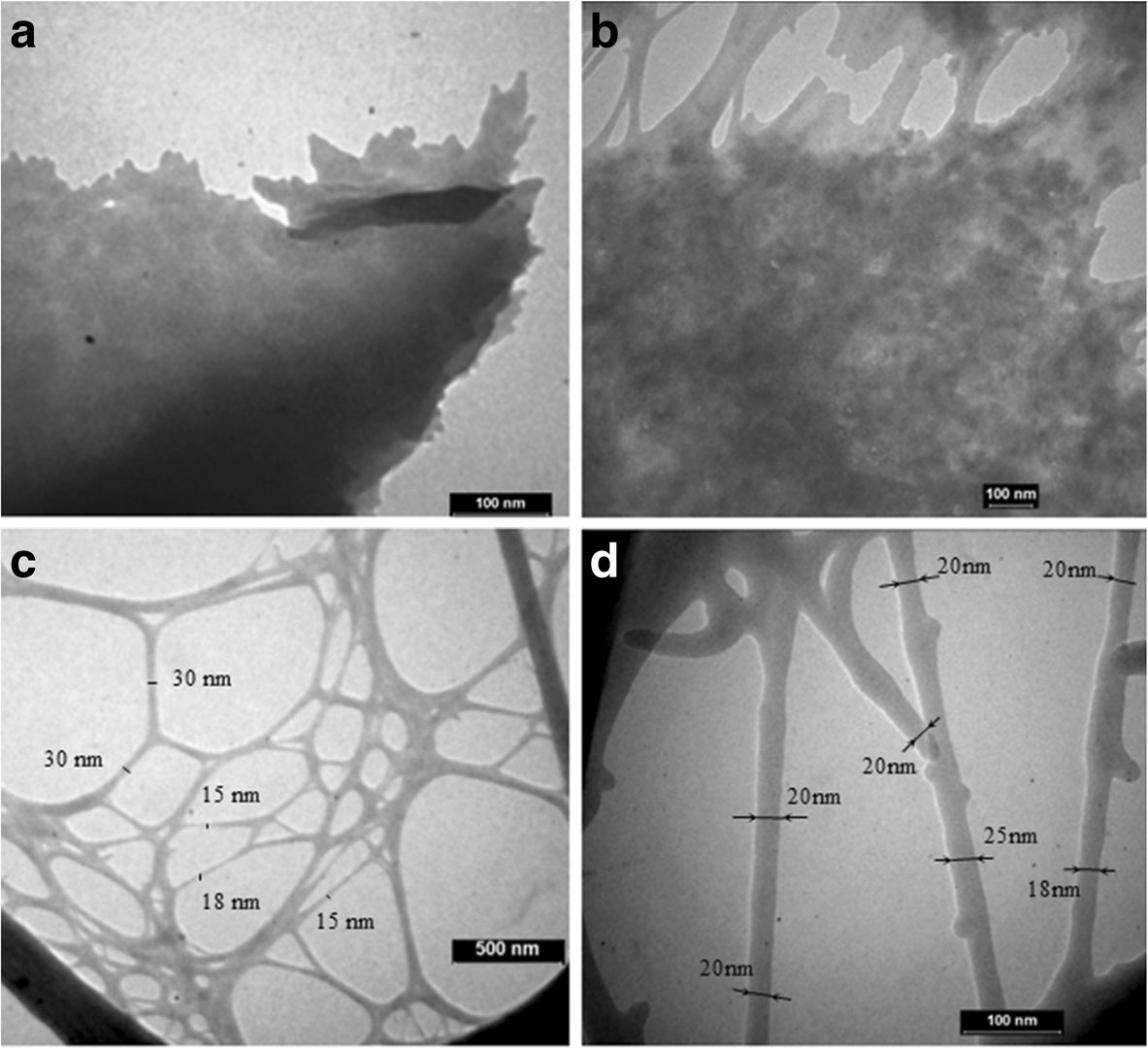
TEM images of the nanocellulose samples after different conditions of hydrolysis with sulfuric acid and ultrasound treatment for 30 min: 30 % at 20 °C, 30 min reaction time (a); 64 % at 20 °C, 5 min reaction time (b); 43 % at 45 °C, 45 min reaction time (c, d)
